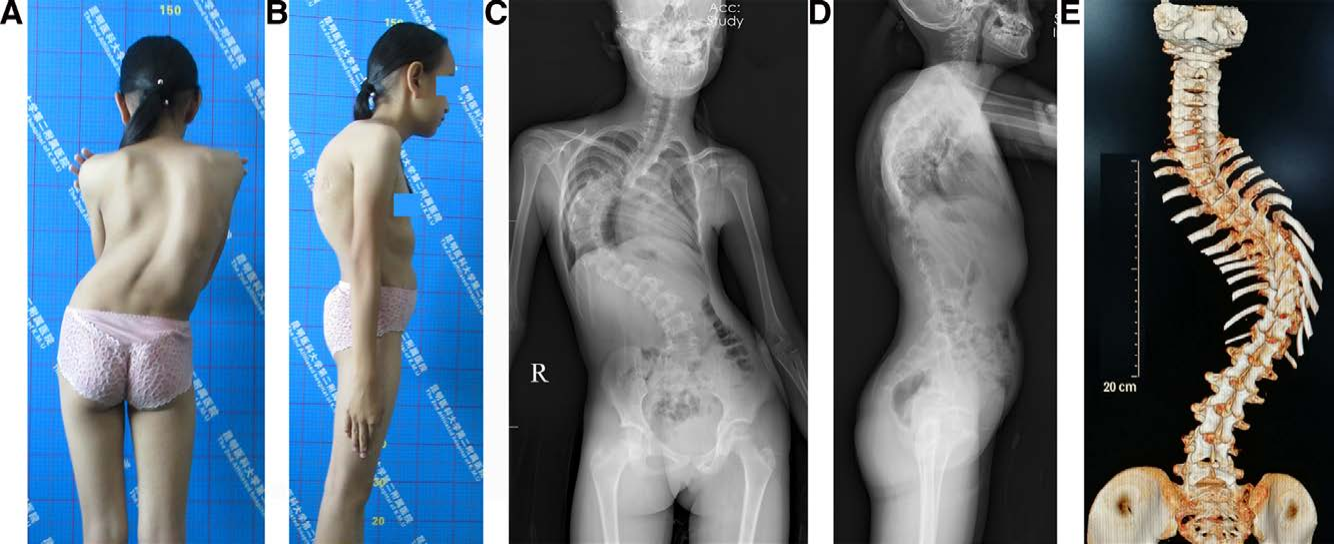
An 11-year-old female patient suffers from severe rigid scoliosis with trunk imbalance. (A) Preoperative posterior view of deformity. (B) Lateral view. (C) A preoperative anteroposterior (AP) X-ray shows a Cobb angle of 112°, radiographic shoulder height (RSH) of 3.4 cm, C7-central sacral vertical line (C7-CSVL) of 2.7 cm. (D) On a lateral X-ray, the Cobb angle is 80°, and the sagittal vertical axis (SVA) is 2.7 cm. (E) Preoperative 3-dimensional CT image reconstruction showing a posterior view of the spinal deformity. AP = anteroposterior, C7-CSVL = C7-central sacral vertical line, CT = computed tomography, RSH = radiographic shoulder height, SVA = sagittal vertical axis.
